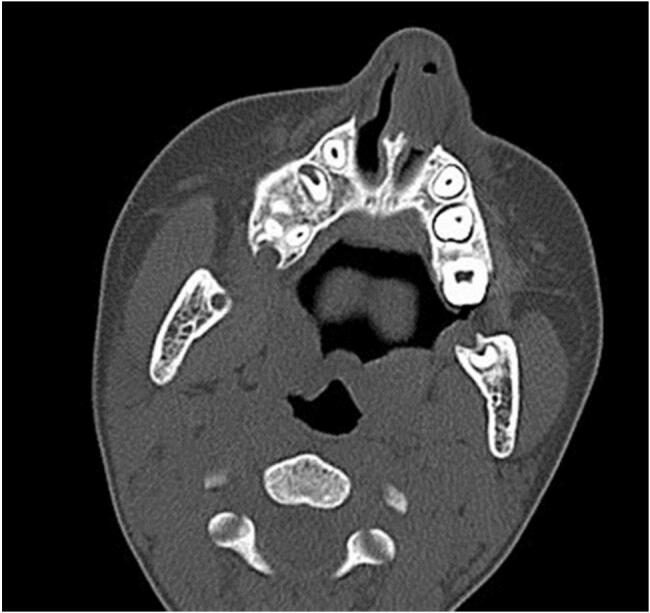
CT-scan (Axial Cut) bony window showed opacity in the left side of the nasal cavity without causing any bone erosion.
